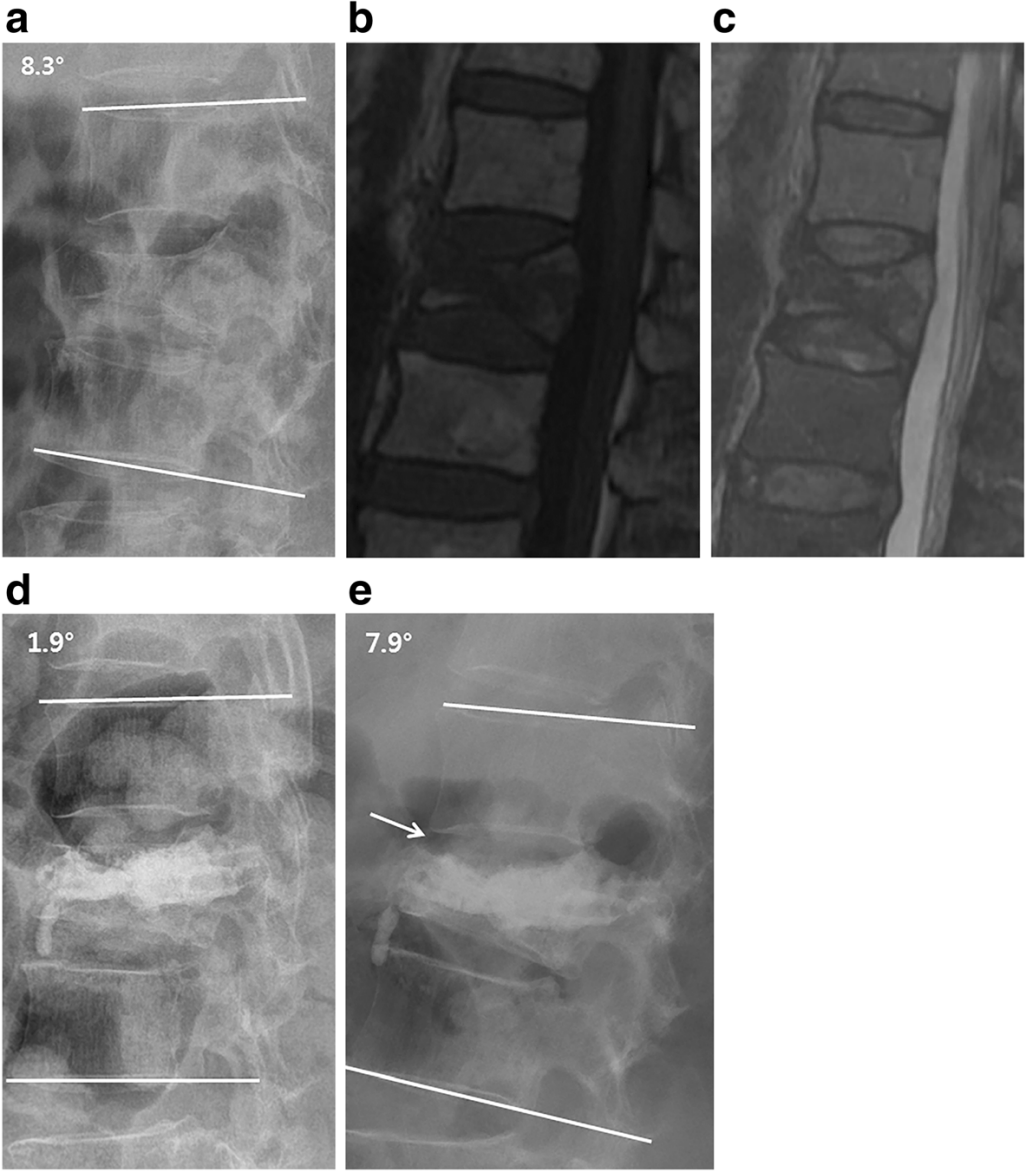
A 70-year-old female patient visited for the lumbago she experienced after she slipped at home. There was a suspected L1 vertebral body fracture based on the lateral plain radiograph (a). A recent fracture in T1WI and T2WI (b, c) was confirmed based on the MRI. After KP, the vertebral height was restored up to 67 % from 45 % before the procedure and the local kyphotic angle was improved from 8.3 ° down to 1.9 ° (d). However, at 1-year follow-up (e), osteolysis (*arrow*) was found around the cement inserted. The vertebral height reduced by 54 % and the local kyphotic angle worsened up to 7.9 ° compared with post-operational figures
